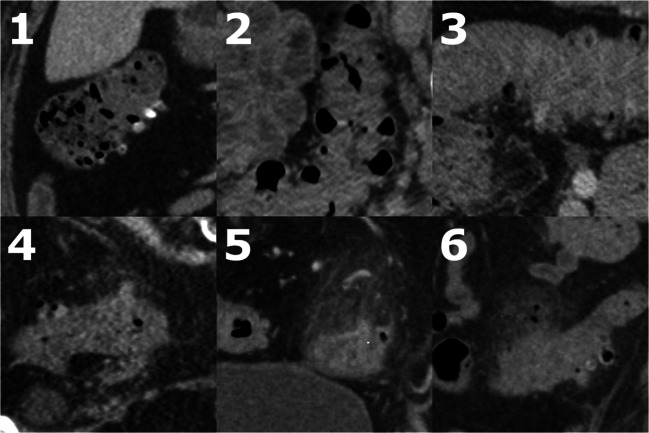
6-level scale used for diverticulitis, with examples from CT scans of this study. 1 Definitely no diverticulitis, e.g., diverticulosis with no wall thickening or fat standing. 2 Unlikely to be diverticulitis, e.g., diverticulosis with wall thickening but no fat stranding. 3 Indeterminate for diverticulitis, e.g., diverticulosis with a long segment of wall thickening and fat stranding. 4 Likely diverticulitis, e.g., diverticulosis with a short segment of wall thickening and mild fat stranding. 5 Definitely diverticulitis, e.g., diverticulosis with short segment of wall thickening and marked fat stranding. 6 Diverticulitis with perforation, e.g., same as 4 or 5 but with extraluminal gas or fluid collection
